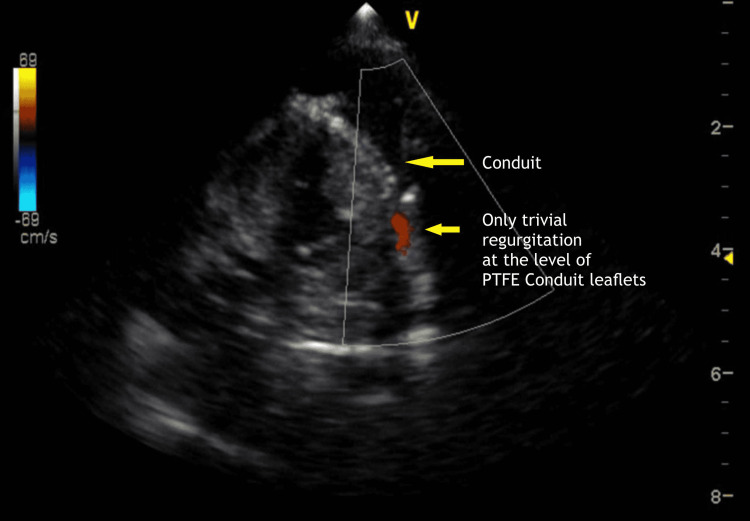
Postoperative 2D echocardiography showing trivial pulmonary regurgitation across the PTFE conduit leaflet PTFE: polytetrafluoroethylene
